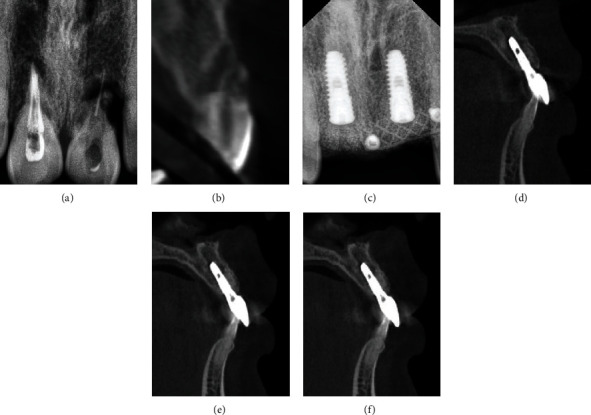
Radiographic findings. (a) X-ray before implantation. (b) CBCT before implantation. (c) 6 months after implantation. (d) Permanent restoration. (e) 1year after restoration. (e) 2 years after restoration.
